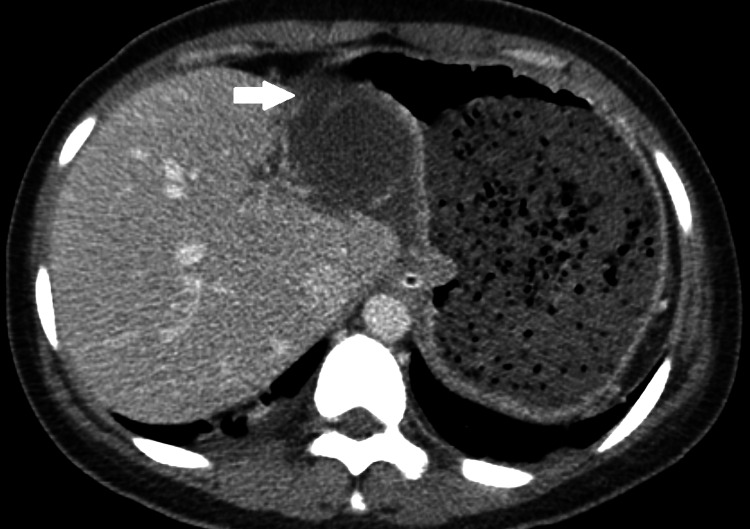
Axial CT scan in venous phase shows diminished enhancement of the gallbladder wall (arrow)
